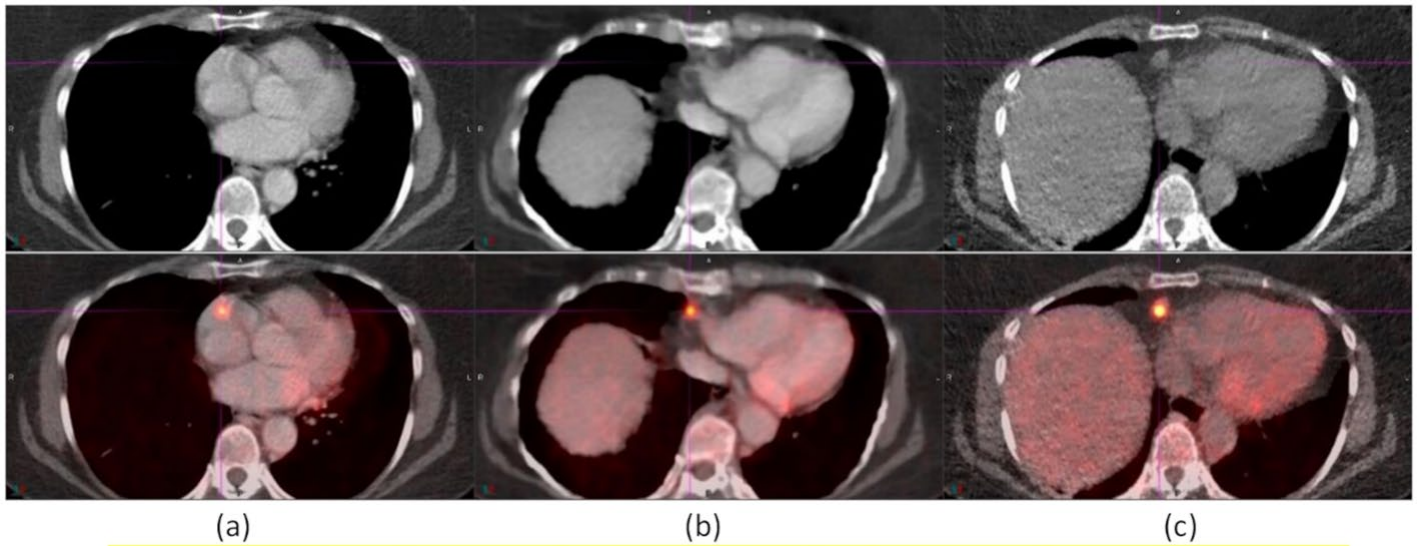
A 1.4-cm para-cardiac lymph node was registered with DDG CT (c) but not with baseline (a) or AIR (b). The SUV_max_ values of the lymph node were 10.78 for baseline, 12.43 for AIR, and 15.41 for DDG CT
